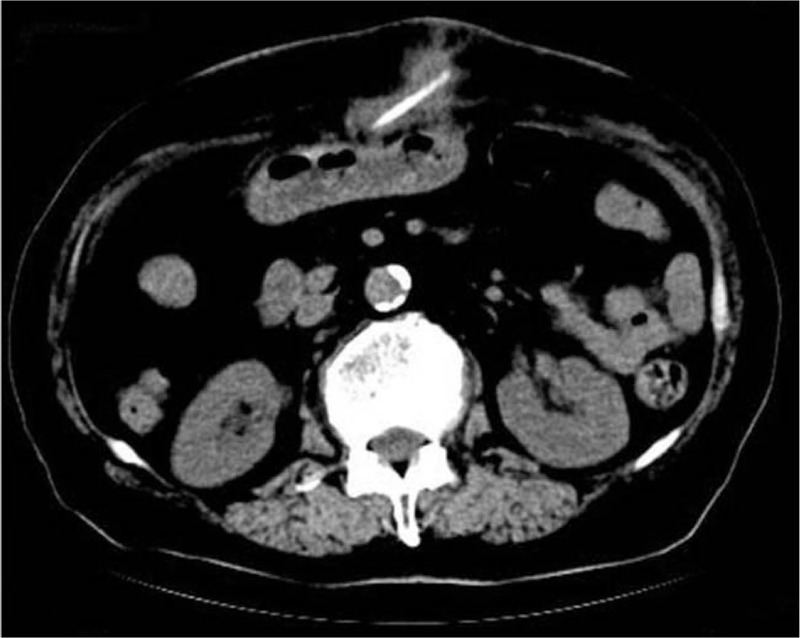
Computed tomography (CT) of the abdomen. The CT scan showed a flaky, soft tissue density shadow between the anterior abdominal wall and subcutaneous fat, and a thin strip of bone-like hyperdense shadow.
